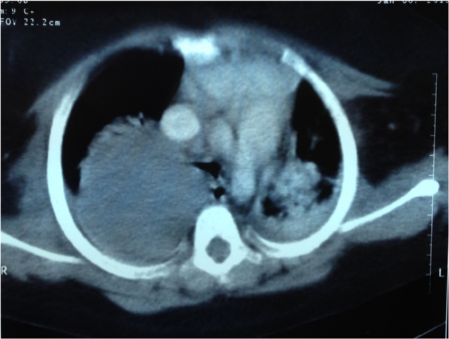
Chest computed tomography scan showing a mass in the right lung
